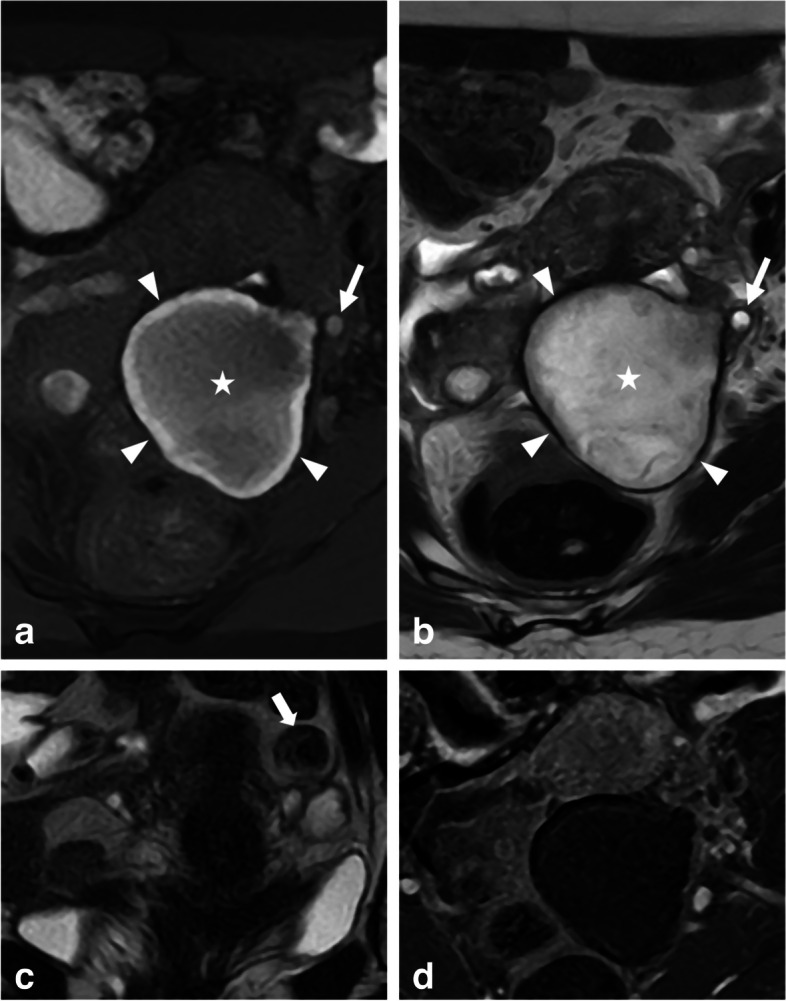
Ovarian severe ischemic necrosis, explored 1 month after presumed acute pelvic infection, in a 47-year-old woman. a Axial T1-W fat-suppressed MR image shows a T1-hyperintense left ovary (star) with a peripheral hyperintense rim (arrowheads), consistent with hemorrhage. A hemorrhagic follicle of the external cortex of the left ovary is identified (arrow). b Axial T2-W MR image shows enlarged centrally migrated left ovary (star) with peripheral hypointense rim (arrowheads). c Coronal T2-W MR image shows a pedicle twist (arrow). d Axial T1-W contrast-enhanced subtracted MR image shows complete absence of enhancement in the left ischemic ovary
